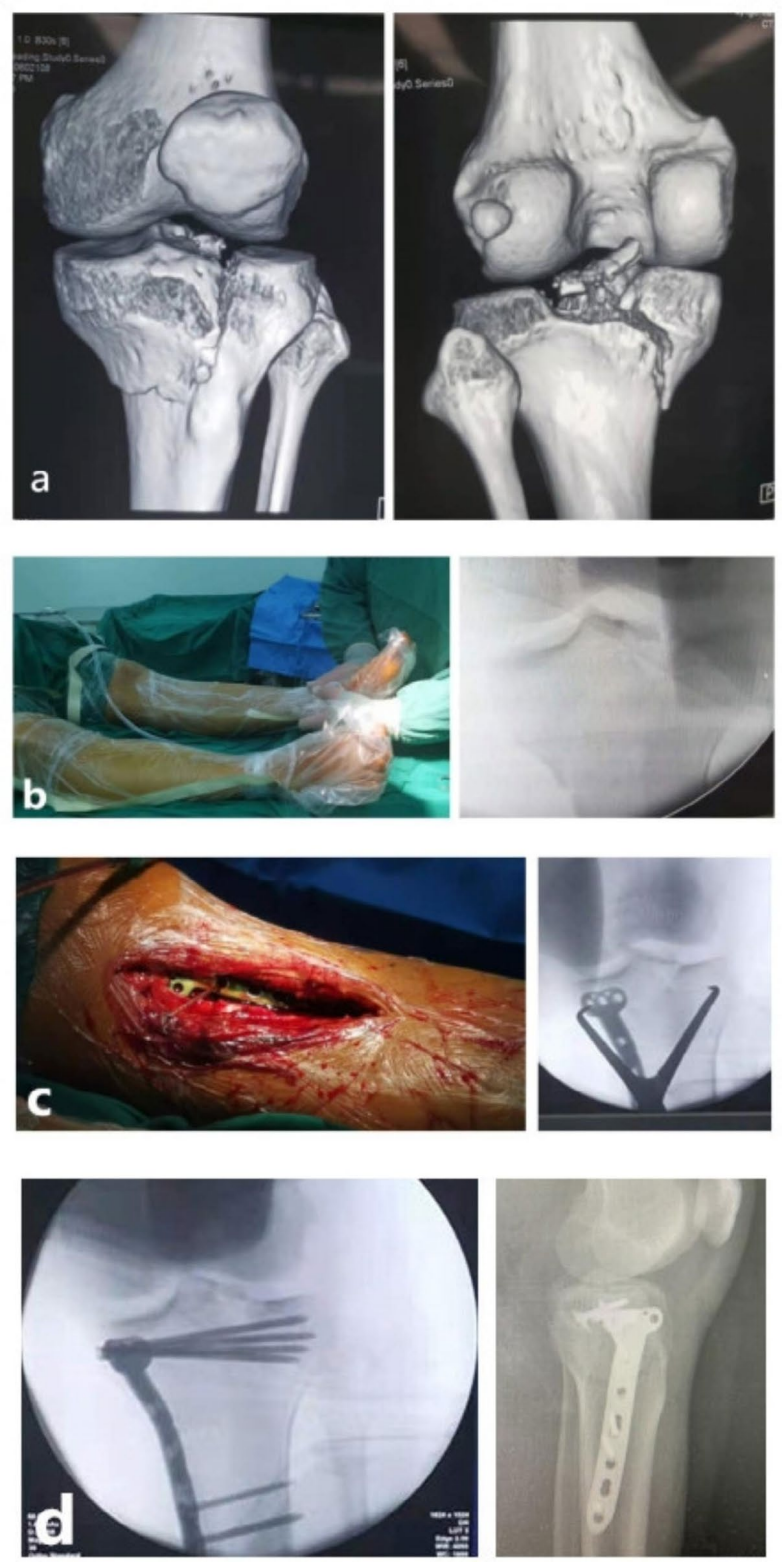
A typical case of a 46-year-old male with Schatzker IV tibial plateau fractures (extension-valgus type). (a) The features of this type were shown in CT images. (b) For the extension-valgus type, the knee should be placed into extension and perform traction for the affected limb. Intraoperative fluoroscopy showed that the dislocation was nearly corrected. (c) After anatomic reduction and temporary fixation, the steel plate was placed upon MFA. Then the patient was turned from a supine position to a prone position and a posterior median incision was made. The posterior intercondylar spine fracture was fixed with screws. (d) The anteroposterior and lateral plain radiographs after the operation were shown
